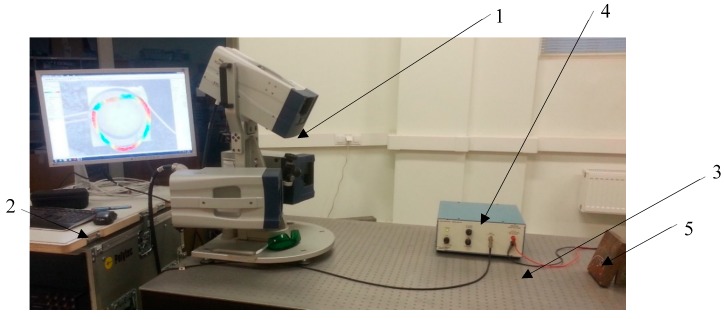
Experimental setup with Polytec Laser Doppler Scanner. 1—PSV-500 3D scanning Head of the vibrometer; 2—PSV-500 3D scanning vibrometer and PC; 3—optical table; 4—amplifier EPA-100 (PiezoSystems Inc., Hopedale, MA, USA); 5—Steel plate with the mounted piezoelectric ring. The piezoelectric ring is mounted on a metal plate and fixed with double-sided adhesive tape.
